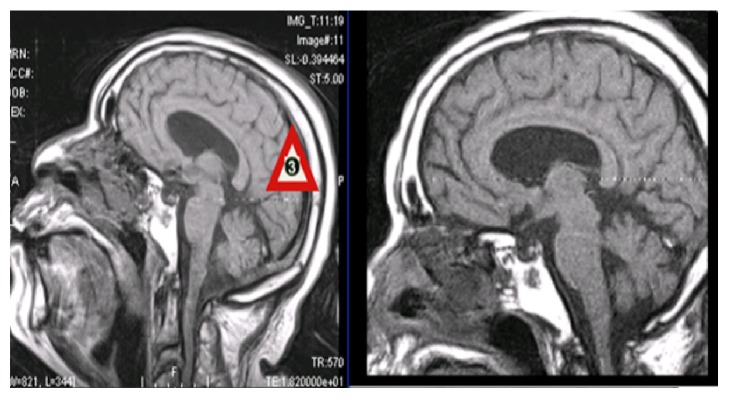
Sagittal T1 diffusion weighted MRI showing a complete opacification of the left sphenoid sinus.
